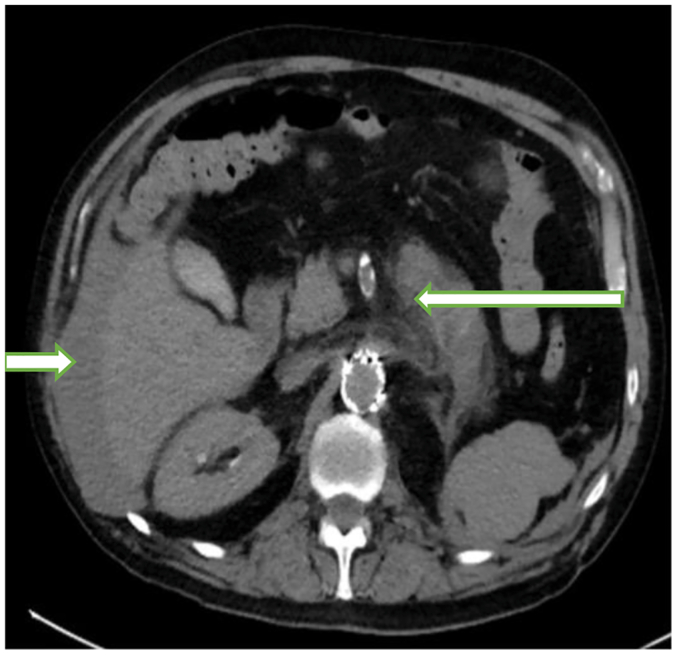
Computed tomography (CT) scan 8 hours later: patient 1.
